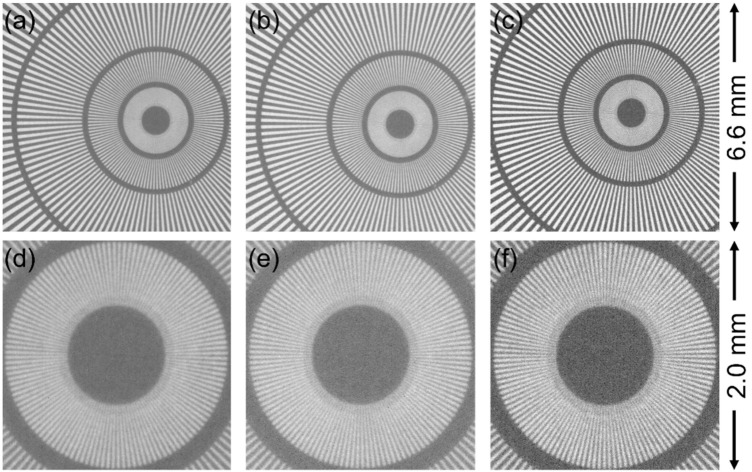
High-resolution neutron radiography taken at 2× magnification under *L*/*D* = 300 conditions by three types of scintillators: (a) an image taken with P43 (10 μm), (b) an image taken with GAGG (100 μm), (c) an image taken with GAGG (10 μm), (d) an enlarged view of (a), (e) an enlarged view of (b), and (f) an enlarged view of (c).
